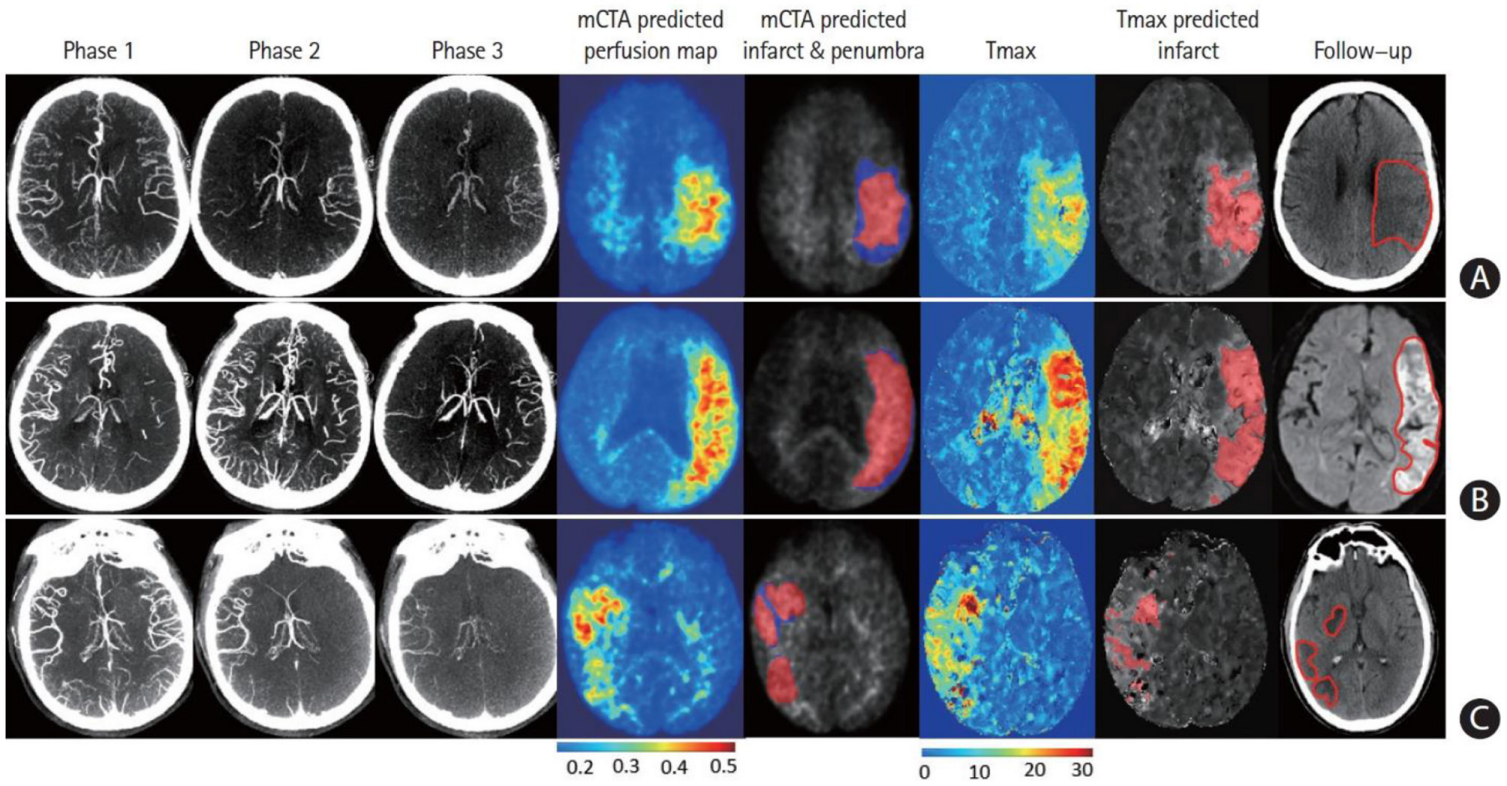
Multiphase computed tomographic angiography (mCTA) predicted an infarct map compared to a computed tomographic perfusion (CTP) time-dependent Tmax threshold map when compared to a follow-up infarct. (A) Patient who achieved reperfusion (mTICI 2b); (B) patient who did not achieve reperfusion; and (C) patient who achieved complete reperfusion with EVT. Columns: mCTA phase 1–3, mCTA predicted perfusion maps, mCTA predicted core (red in column 5) and penumbra (blue in column 5) overlaid on the mCTA predicted perfusion map, CTP Tmax maps, CTP time-dependent Tmax threshold predicted infarct, infarct contoured in follow-up imaging, respectively. The penumbra is shown as affected tissue from the penumbra model minus affected tissue from the core model. [Reprinted with permission from Journal of Stroke, 2021 (36)].
